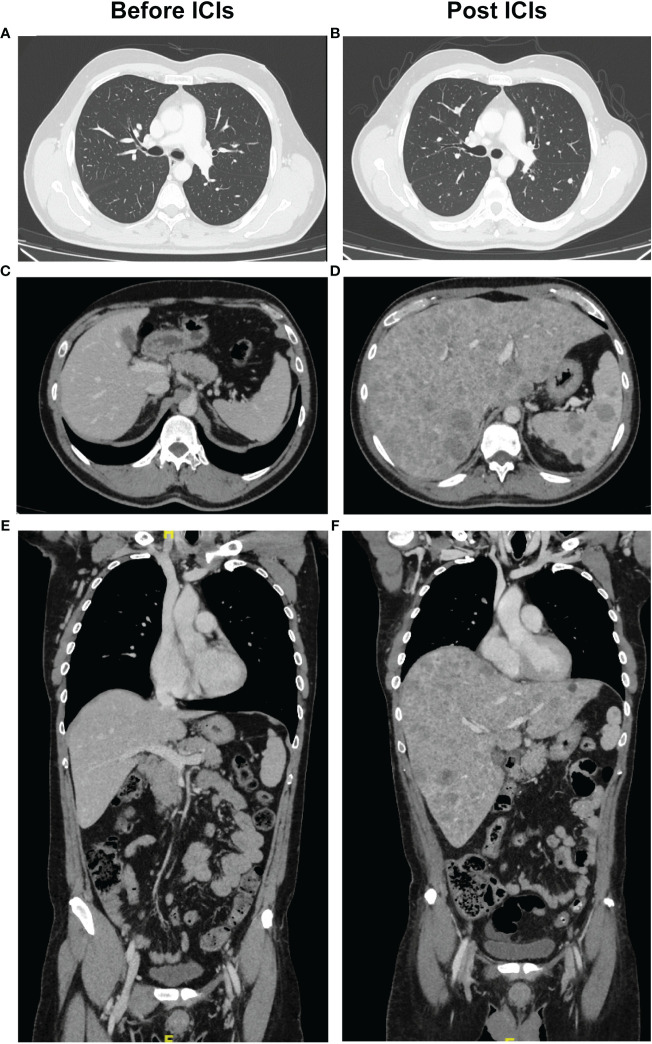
Radiographic evidence of hyperprogression after ICI of the second case. Pre-treatment with ICI, CT scan: (A) chest; (C-E) liver and spleen. Post treatment with ICI, CT scan with evidence of disease progression in the (B) chest and (D-F) liver and spleen on transverse and coronal planes.
